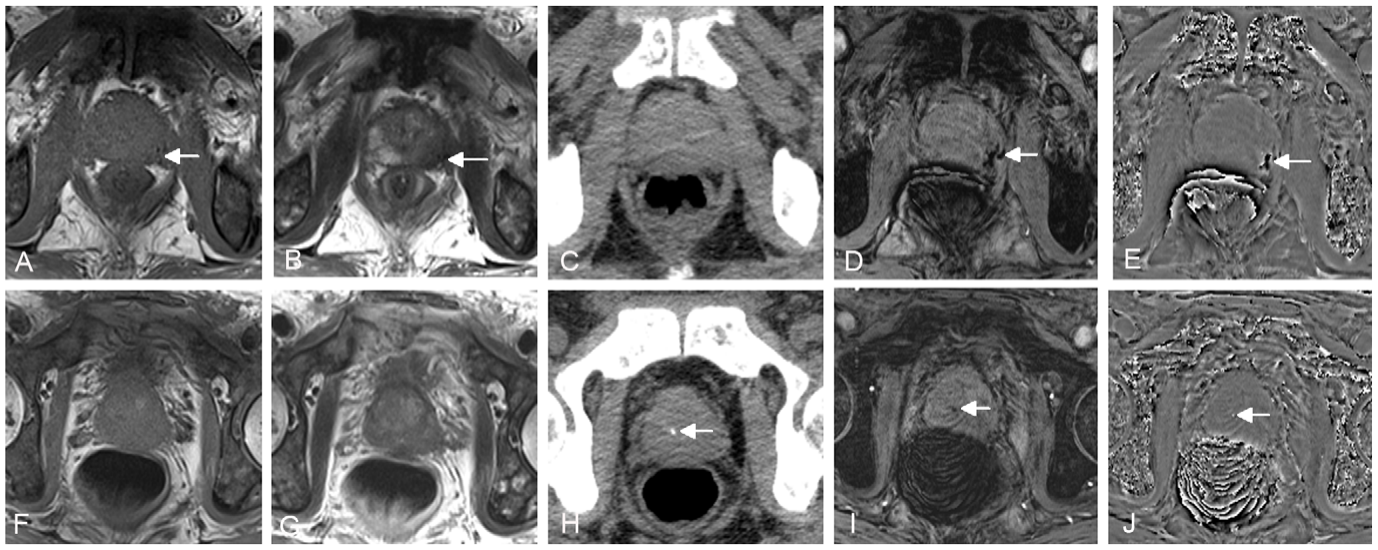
A 66-year-old man with prostate cancer in peripheral zone of the prostate. Low signal on conventional T1WI (A) and T2WI (B) (arrows) indicates tumor hemorrhage. No hemorrhage is demonstrated on CT (C). The tumor hemorrhage was also seen with SWI (D) and filtered phase image (E) (arrows). The images in second row come from another slice of the same patient. No prostatic calcification is demonstrated on conventional T1WI (F) and T2WI (G), but dot-like high density on CT (H), low signal on SWI (I) and high signal on filtered phase image (J) (arrows) indicates calcificaiton.
